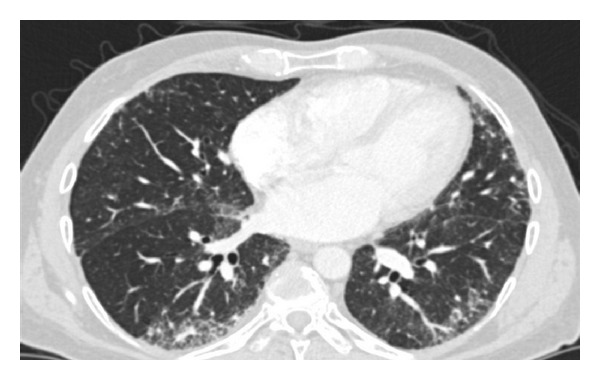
Chest CTA showing subpleural pulmonary fibrosis, inter- and intralobular septal thickening, architectural distortion with traction bronchiectasis and bronchiolectasis, and distortion of interfaces as well as diffuse prominence of the segmental and subsegmental pulmonary arteries with extension of vessels to the lung periphery.
